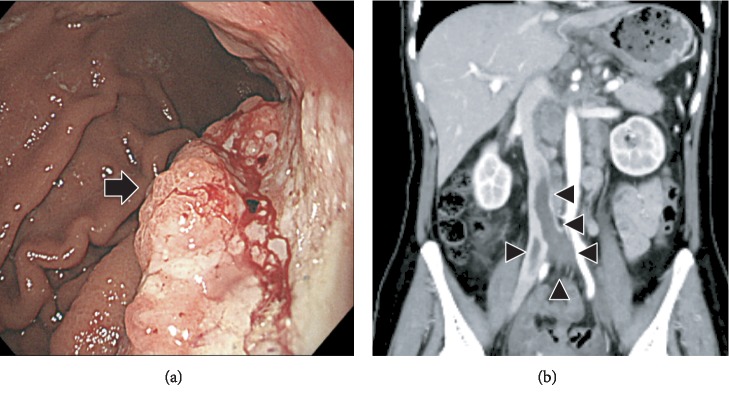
(a) Gastroscopy showing elevated lesions with bleeding in the body of the stomach (arrow). (b) Whole-body CT showing a large thrombus extending from the bilateral common iliac veins to the inferior vena cava (arrowhead).
